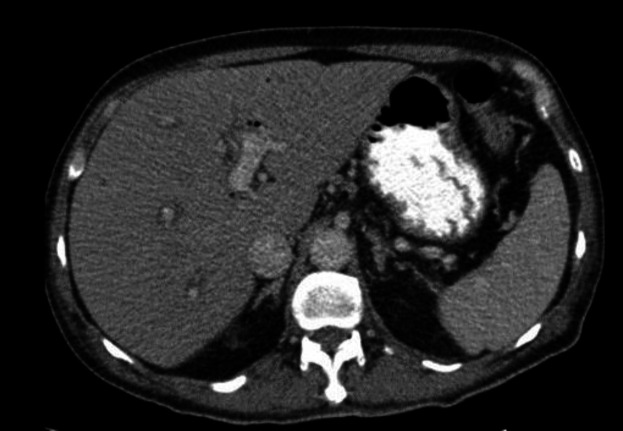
The CT scan showed evidence of pneumobilia in biliary ducts
